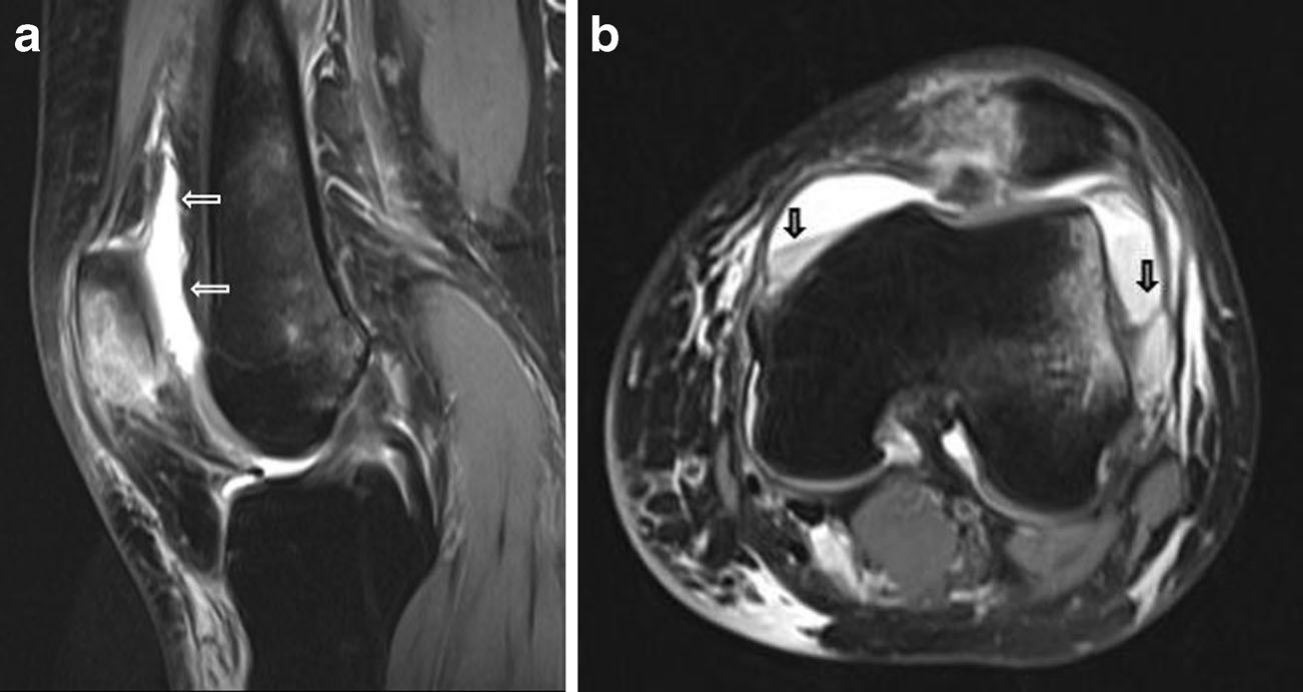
HFP acute trauma (direct contusion). MRI proton density with fat saturation images show HFP oedema, patellar oedema and joint effusion (*thin arrows*) (a) with fluid-fluid levels in axial image (*wide arrows*) related to hemarthrosis (b)
